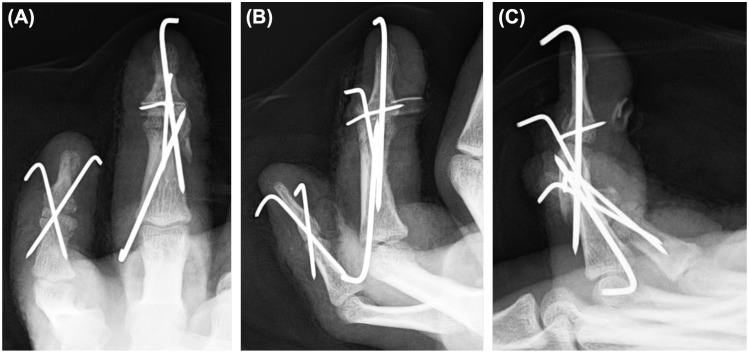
Immediate postoperative radiographs of the left ring and little fingers. The ring finger was stabilized using transarticular DIP joint pinning, extension-block pinning, additional K-wire fixation of the dorsal base fragment, and pull-out fixation of the tendon-attached fragment. The little finger was stabilized with cross K-wire pinning. (A) Posteroanterior view. (B) Oblique view. (C) Lateral view.
